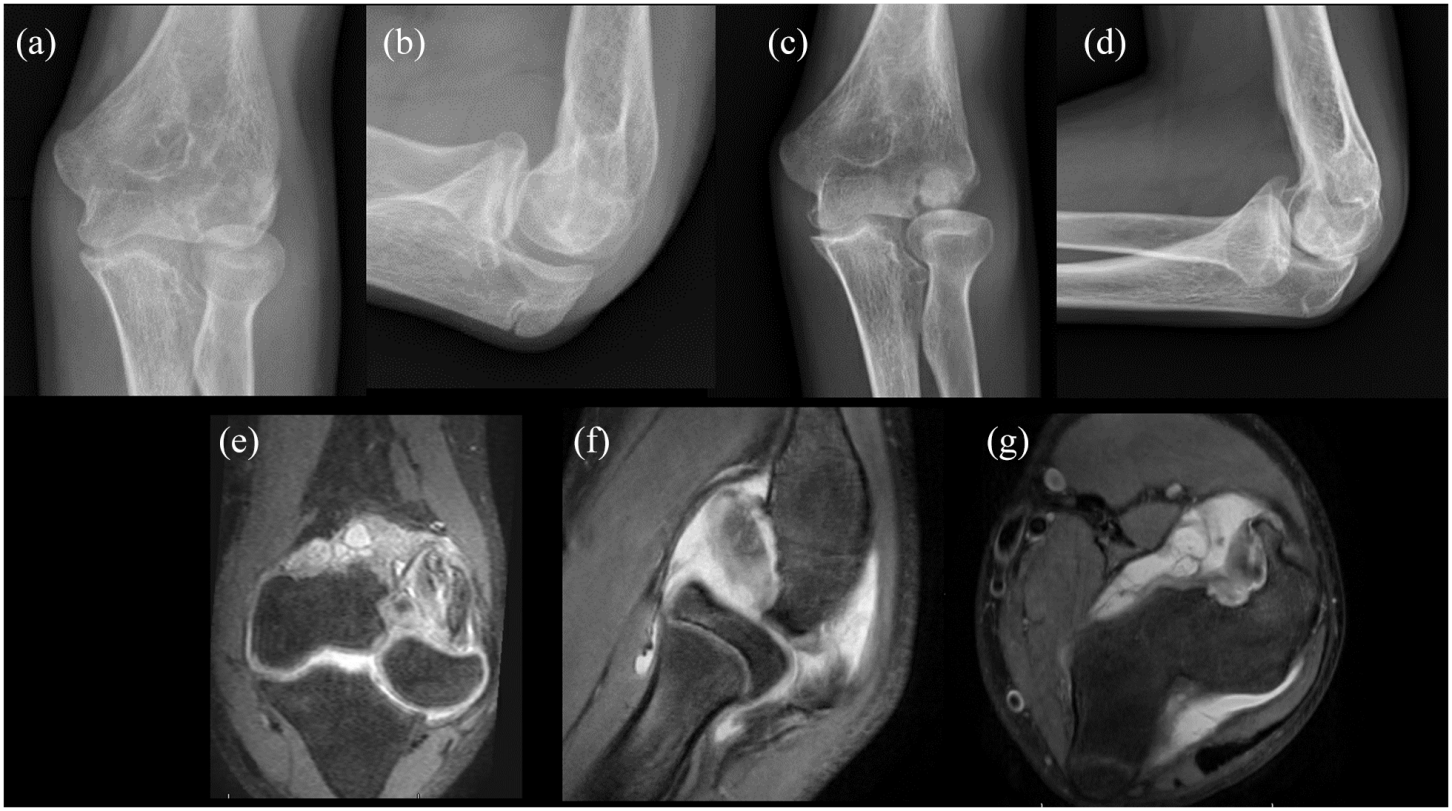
Pre- and post-operative imaging of osteochondral allograft implantation of 14-year-old male patient with a large osteochondral defect (22 mm × 22 mm). Pre-operative radiographs (a and b) and post-operative radiographs (c and d) demonstrate interval placement of an 18 mm × 18 mm osteochondral allograft. Pre-operative MRI (e, f, g) demonstrates the size of the capitellar osteochondritis dissecans.
